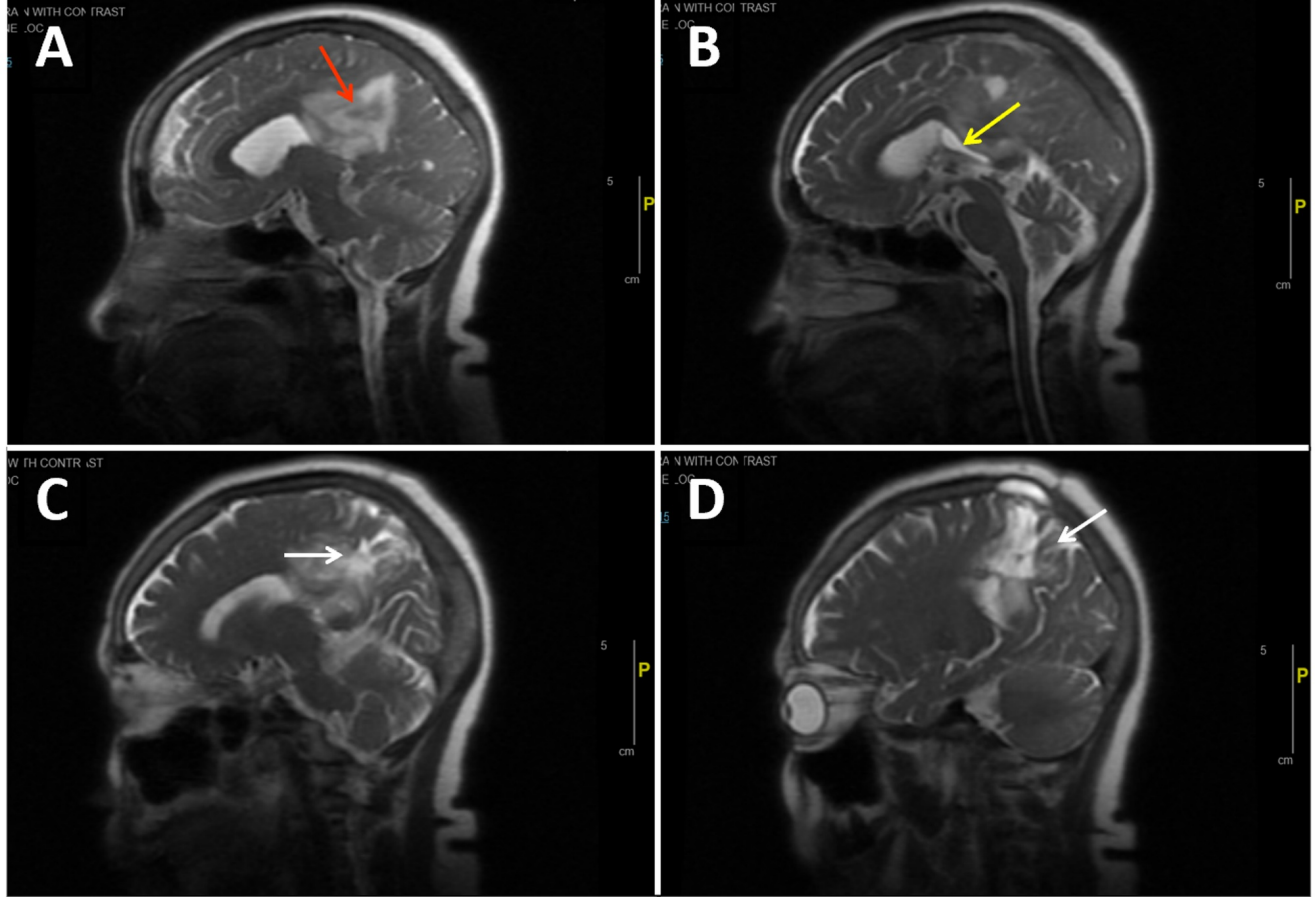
Magnetic resonance imaging of the brain A: large hemorrhage in the cingulate gyrus (red arrow); B: mass effect compresses the corpus callosum (yellow arrow); C and D: a large amount of vasogenic edema extends into both parietal lobe white matter (white arrows).
